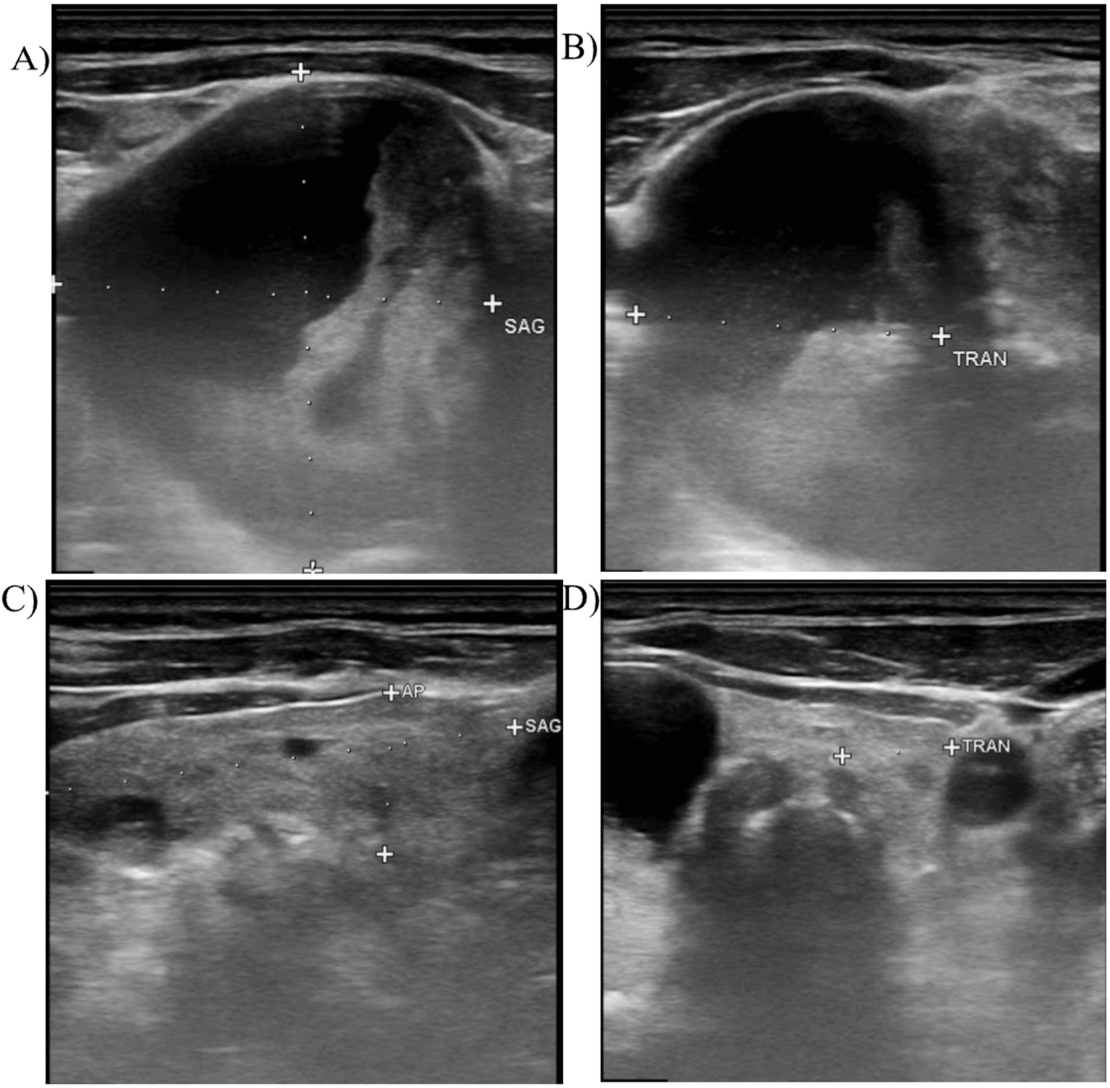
Sonograms of right and left lobes A and B depict the right lobe, C and D depict the left lobe. B and D depict the right and left lobes in a transverse view, respectively, and A and C depict the right and left lobes in a sagittal view, respectively.
